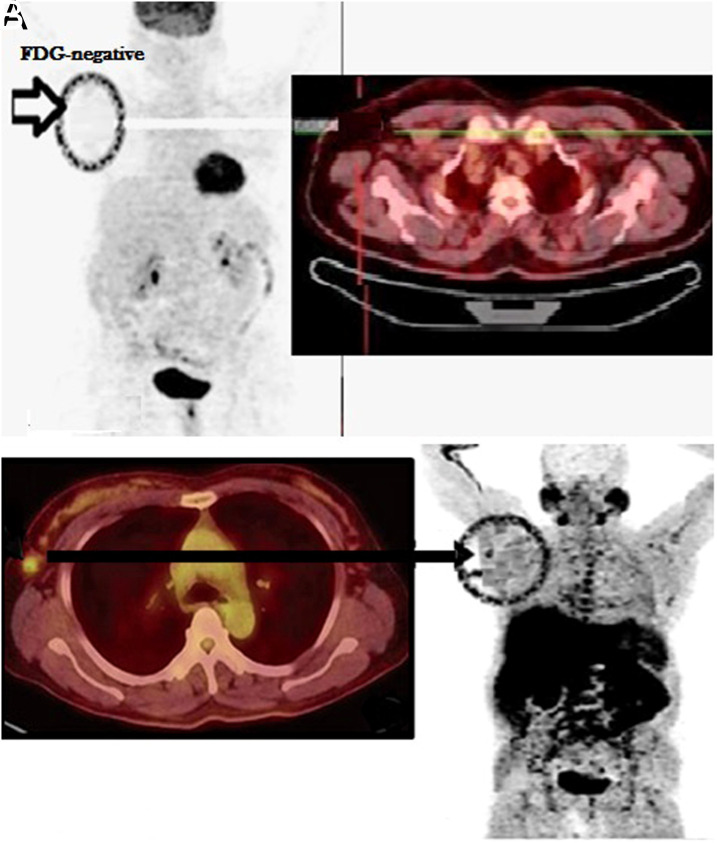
A 51-year-old lady with single ER/PG human epidermal growth factor receptor 2 negative. Coronal (A) MIP positron emission tomography (PET). Fluorodeoxyglucose-negative right axillary lymph node deposit. Axial (B) fused PET-computed tomography (CT): Fluorocholine (FCH)-positive right axillary lymph node. The added value of 18F FCH-avid right axillary lymph node is its higher sensitivity than 18[F]-FDG in a small nodal metastasis deposit.
